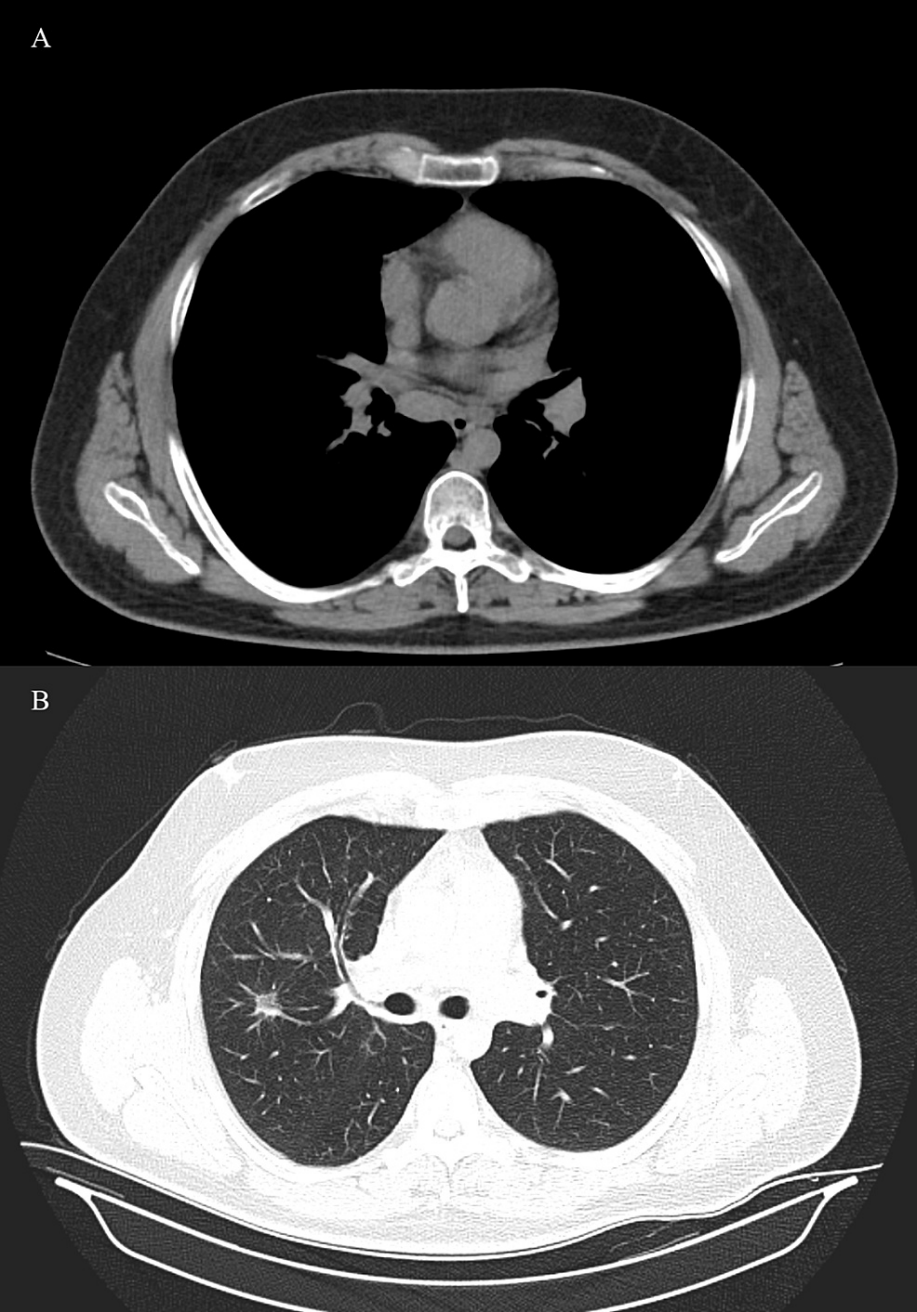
Thoracal CT shows the regression in the size of the nodules in the lungs (A) and the mediastinal lymphadenopathies (B) after high-dose steroid treatment.
